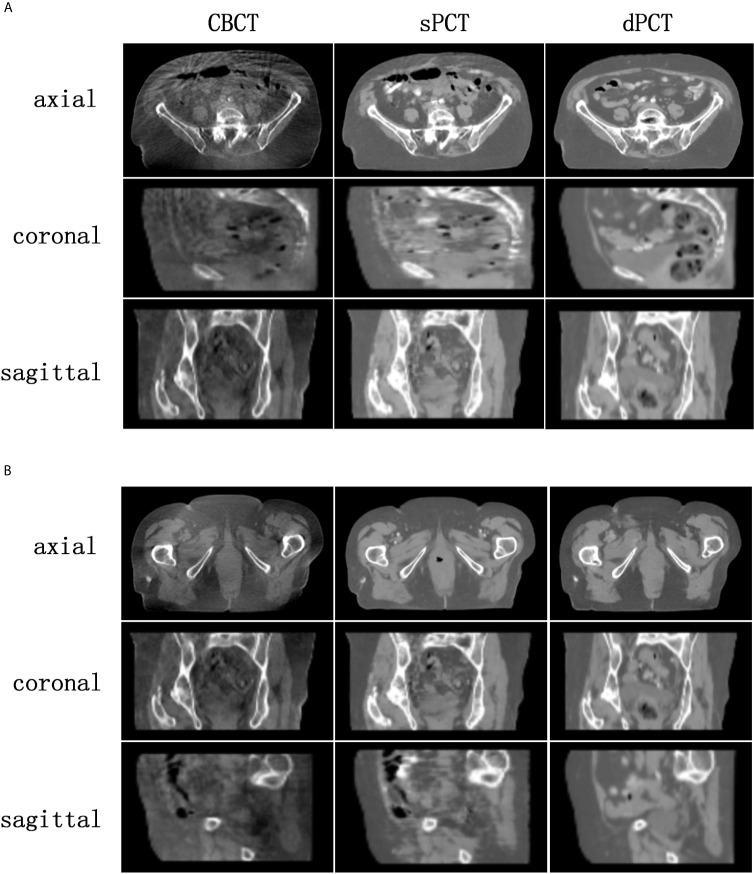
Comparison of image quality among CBCT, sPCT, and dPCT of two patients (A, B). For each patient, the images in the top, middle, and bottom rows are axial, coronal, and sagittal, respectively. The left, middle, and right images represent CBCT, sPCT, and dPCT respectively. The display window is (700, 1300) HU.
